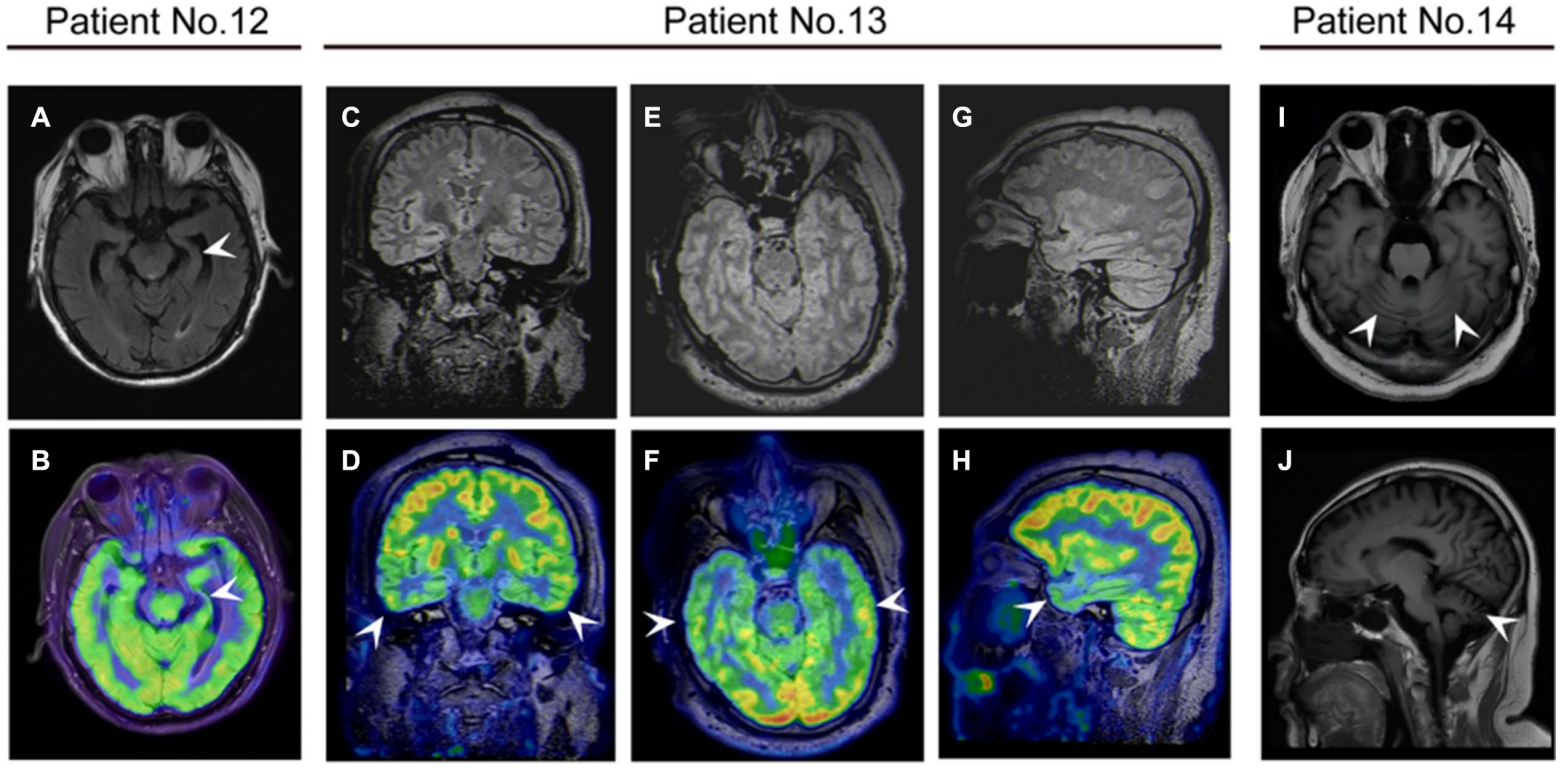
The neuroimaging of patients with anti-DPPX encephalitis. (A,B) Patient 12 with cognitive impairment exhibited atrophy of the left hippocampus on magnetic resonance imaging (MRI) and decreased ^18^F-fluorodeoxyglucose (FDG) uptake in the left hippocampus on positron emission tomography (PET)/MRI. Patient 13 with cognitive impairment exhibited decreased ^18^F-FDG uptake in the bilateral temporal lobes on PET/MRI in (C,D) coronal (E,F) axial, and (G,H) sagittal views. (I,J) Patient 14 with ataxia showed cerebellar atrophy on MRI.
